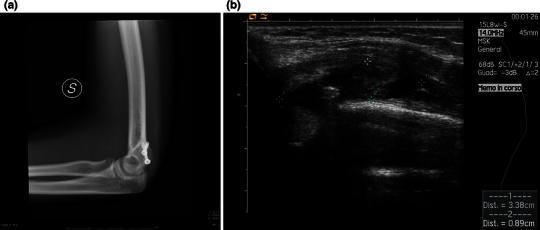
Case 2.a X-ray showing a screw tip slightly protruding posteriorly from the bone surface.b Ultrasound examination showing the presence of a hematoma with a partial lesion of the myotendinous junction of the triceps over the protruding screw tip
